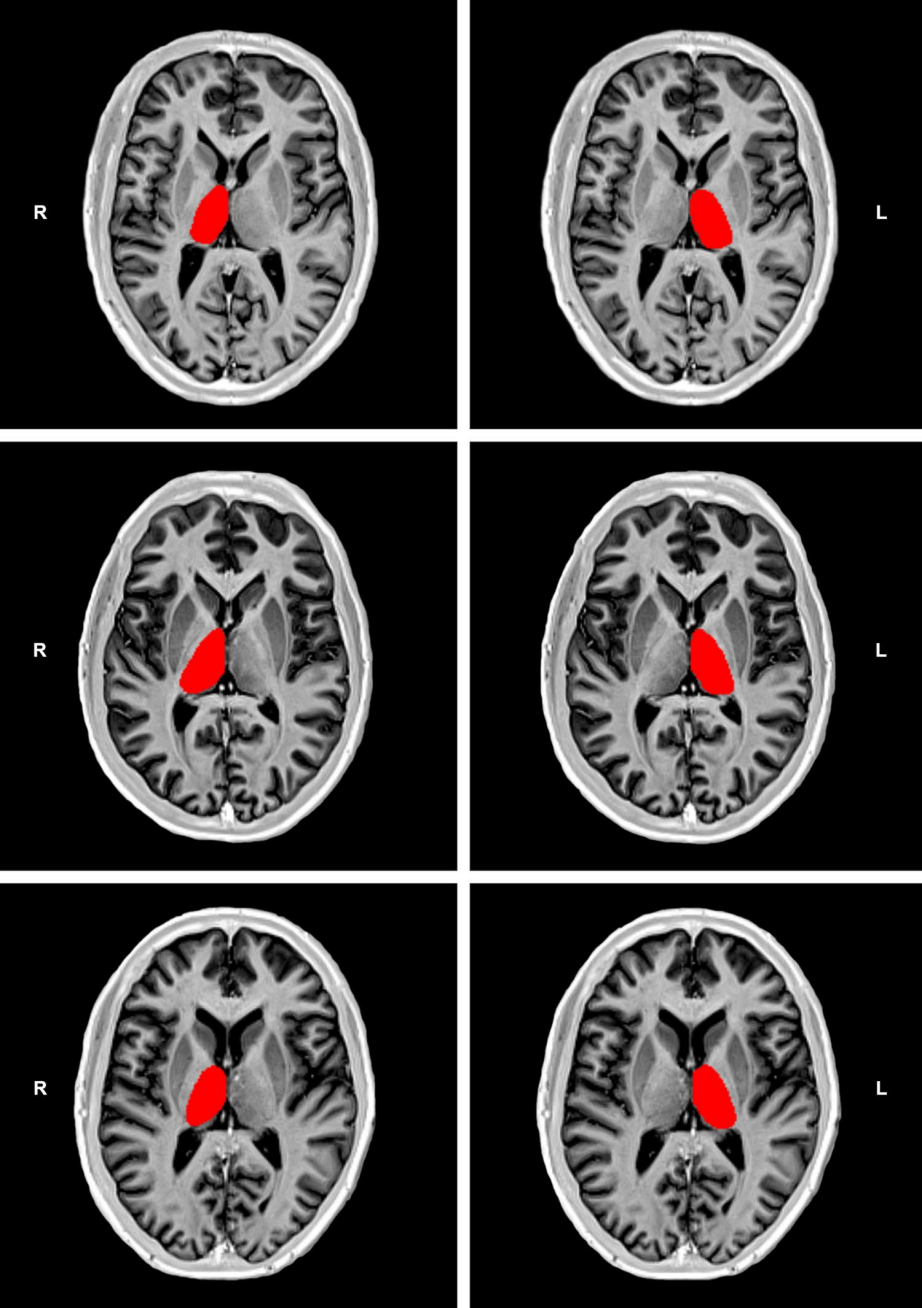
Examples of thalamic segmentation on bias corrected inversion recovery (IR) turbo spin echo (TSE) T1-weighted images (T1-WI) from one patient with nonpainful diabetic peripheral neuropathy (DPN) in the top row and one patient with painful DPN in the middle row. In the bottom row, an example of a similar segmentation procedure on a 46-year-old healthy subject is shown. This example is presented for visualization purposes only, and was randomly selected from a sample of a previous study using similar IR TSE T1-WI to segment deep gray matter structures^11^. Please note the apparent reduction of thalamic volume (especially the thinner cross-sectional thalamic distance) in the patient with nonpainful DPN, especially in the right thalamus, relative to the other examples.
